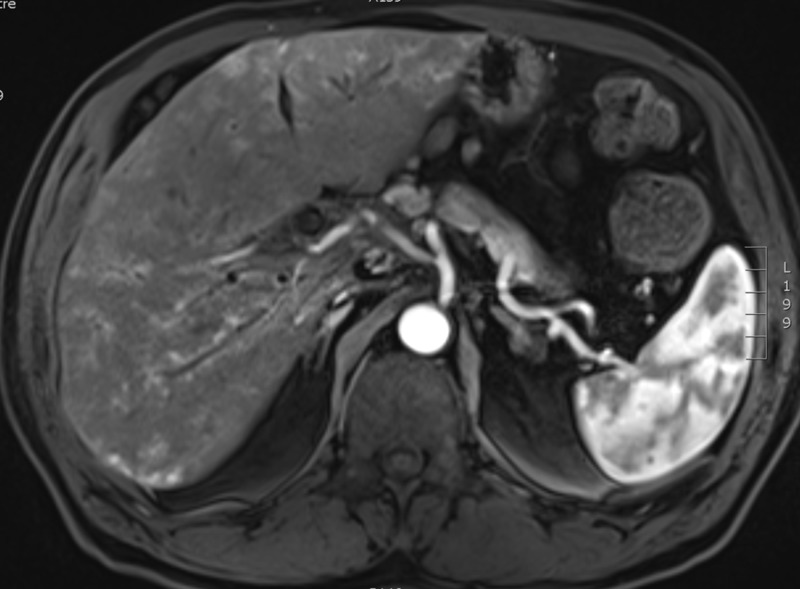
Arterial phase MRI liver (VIBE pulse sequence) obtained four months post-SBRT to the NSCLC demonstrating the complete resolution of the hepatic metastasis. VIBE, volumetric interpolated breath-hold examination.
